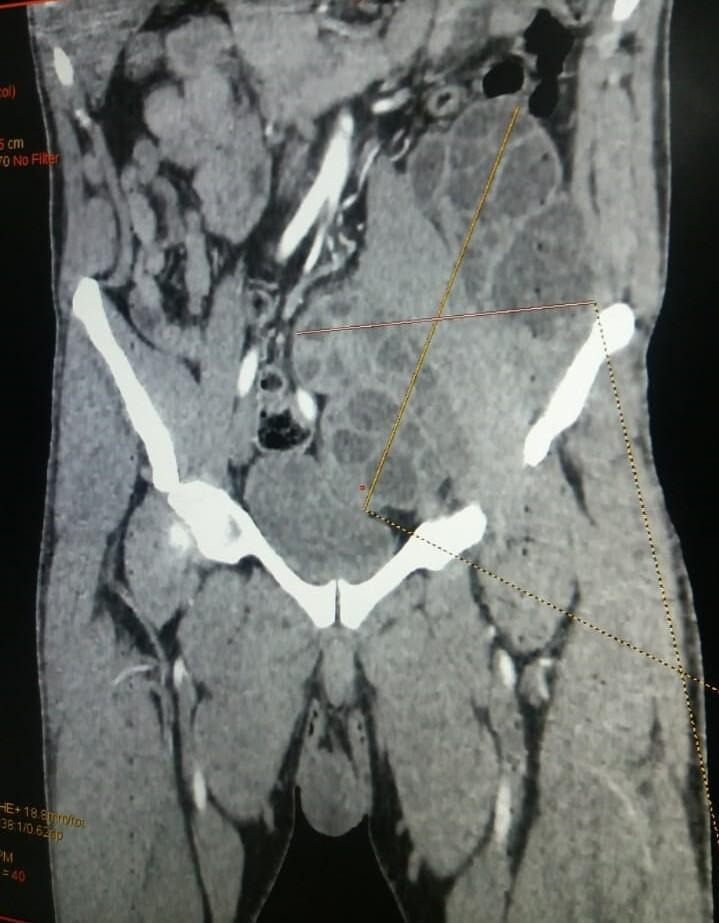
Coupe sagittale de tomodensitométrie injectée abdominopelvienne montrant une énorme masse kystique multicloisonnée évoquant une hydatidose Sagittal section of an injected abdominal-pelvic CT scan showing a huge multiclonal cystic mass suggestive of hydatidosis
